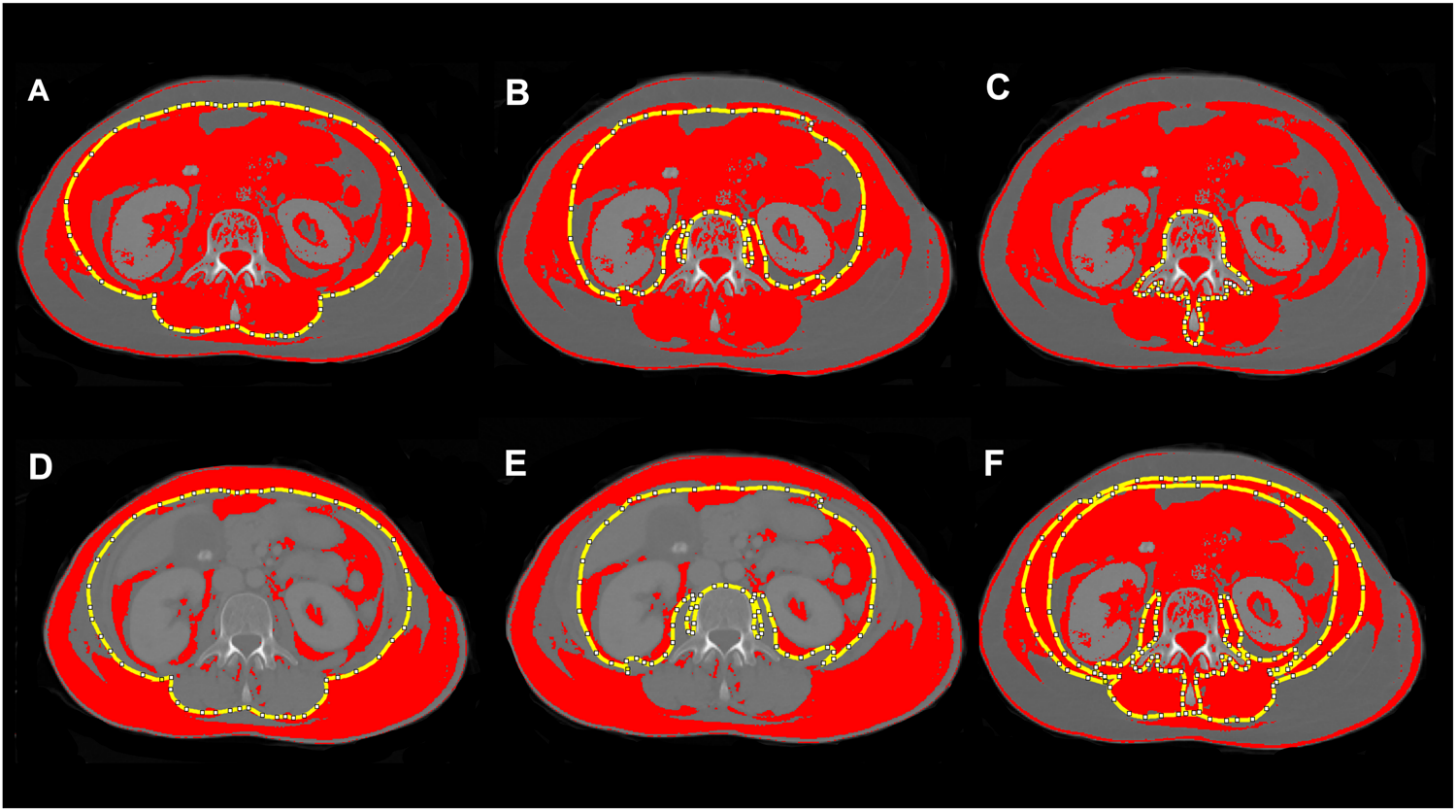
Postprocessing contours to quantify conventional CT body composition parameters. Displayed is the outer (A) and inner (B) perimeter of the abdominal and paraspinal muscle, and of the third lumbar vertebra (C), as well as the whole abdominal muscle area (F) each with the muscle-specific threshold of –29 to +150 HU. With a fat-specific threshold of −150 to −30 HU, (D) displays the outer and (E) the inner muscle perimeter. The skeletal muscle index is then given according to Gomez-Perez et al [23] by subtracting B and C from A. The muscle radiodensity attenuation is given by F. The subcutaneous adipose tissue is given by the area outside the defined muscle perimeter in D, and the visceral fat by the area within the perimeter in E
